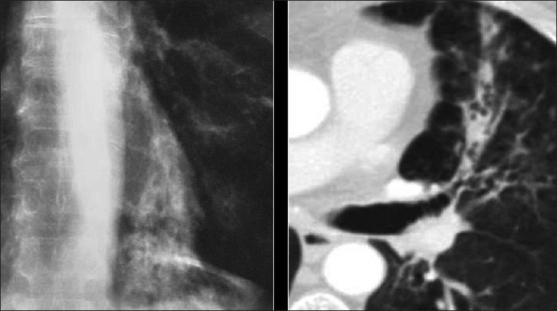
Aspergillosis. ABPA a chest X-ray/computed tomography on the same patient shows bilateral lower lobe consolidation, bronchiectasis and airway impaction or “finger in glove” appearance
